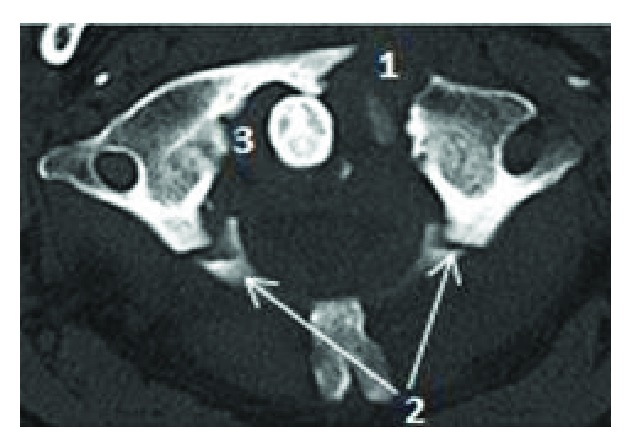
Cervical spine CT scan showing type III Jefferson fracture with anterior arch fracture (1) and posterior arch fractures (2). Note the widening of the atlantodens interval (3).
